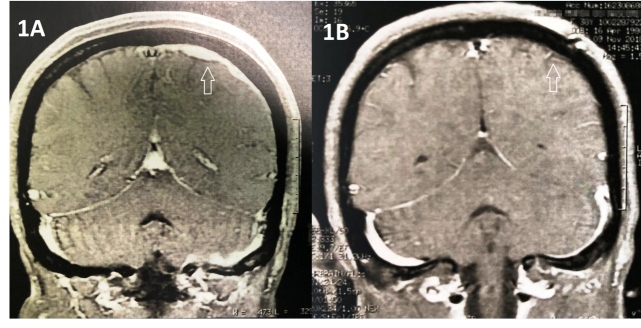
Coronal section; (A) Coronal section showing contrast-enhanced meningeal thickening in the upper left hemisphere on T1-weighted MRI; (B) Control MRI, after clinical treatment, showing radiological improvement in the meningeal thickening.
